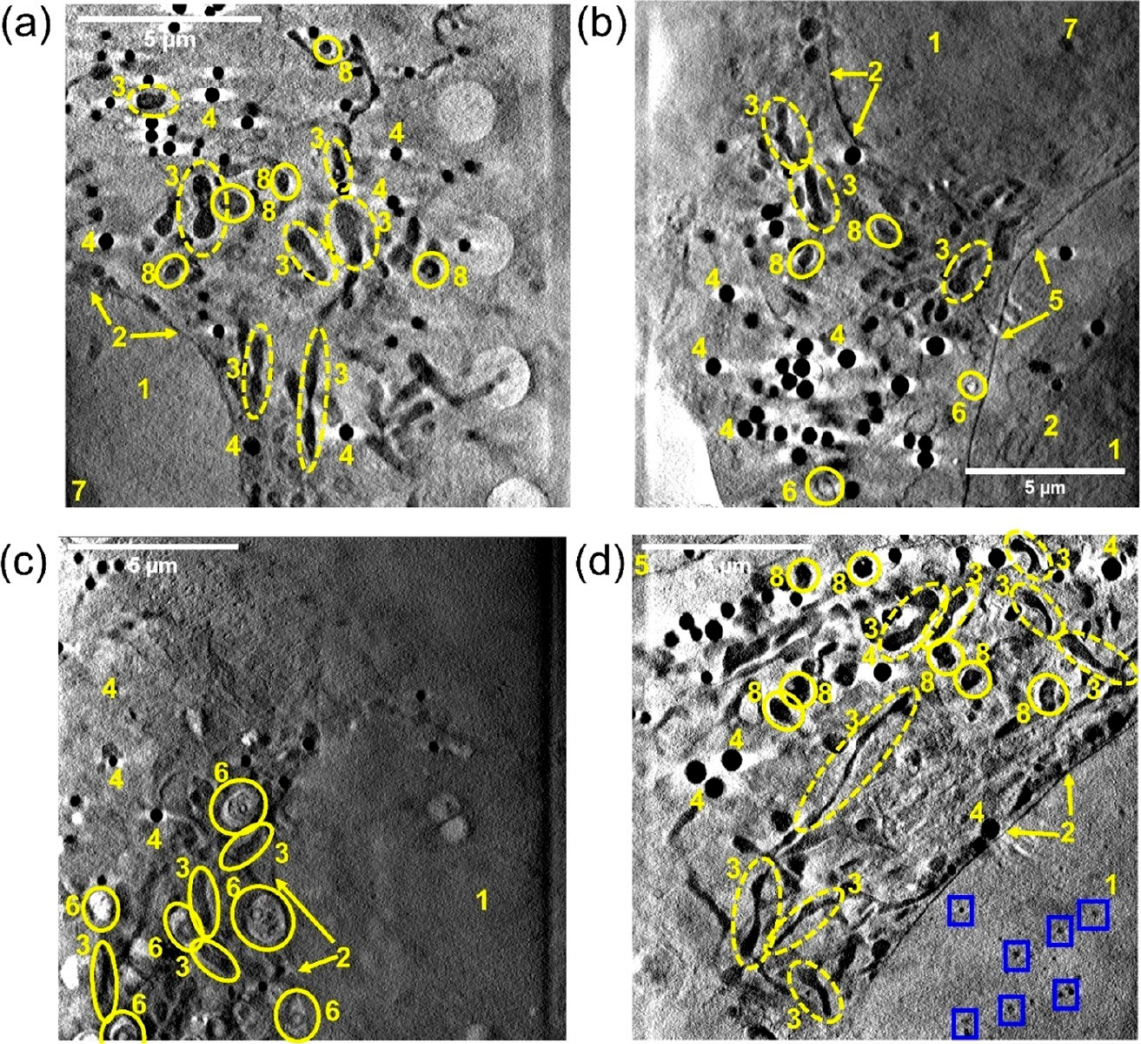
X-ray tomograms of cryopreserved
